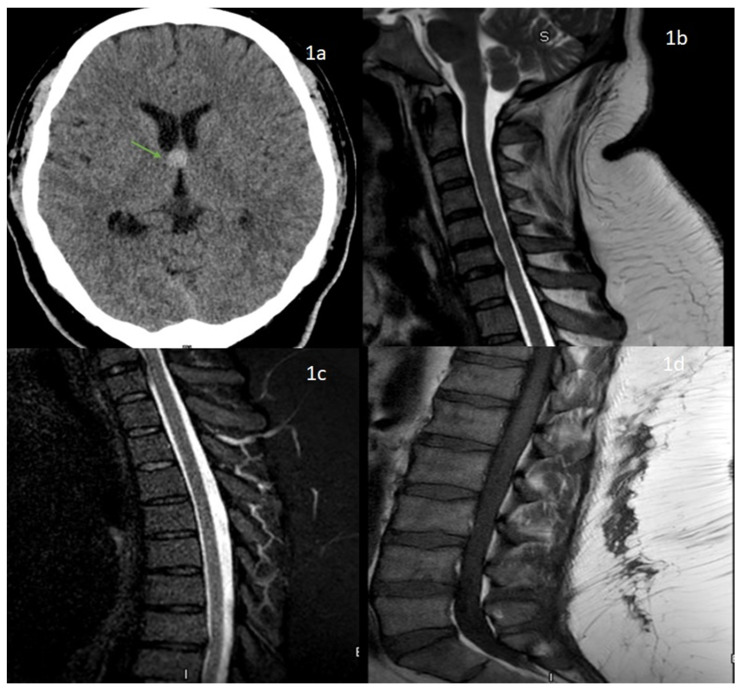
(1a) CT head showing colloid cyst at the level of foramen of monroe (green arrow). (1b,1c) MRI cervical and thoracic spine sagittal images showing no abnormal intramedullary cord signal changes. MRI lumbar spine (1d), T1 weighted sagittal image showing no degenerative disc disease.
